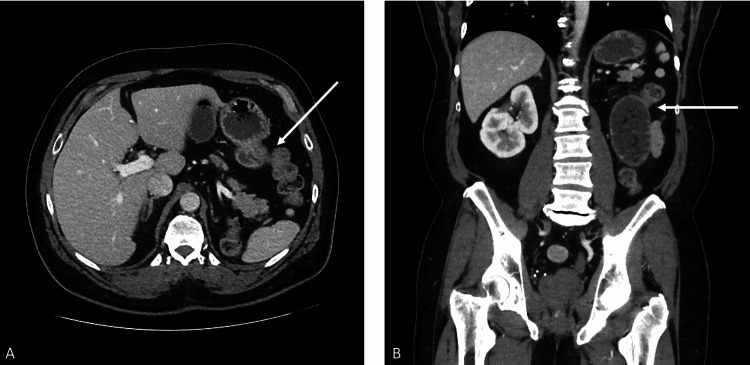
CT of the abdomen without contrast: (A) transverse plane and (B) coronal plane There are moderate recent distended loops of the small bowel from the level of the duodenal C-loop down to the left pelvic inlet. This finding has developed since a previous study and presumably represents a partial small bowel obstruction (arrows). The exact etiology and location of the obstruction are not entirely certain but might be related to adhesion. The more distal small bowel beyond this level is normal in caliber.
